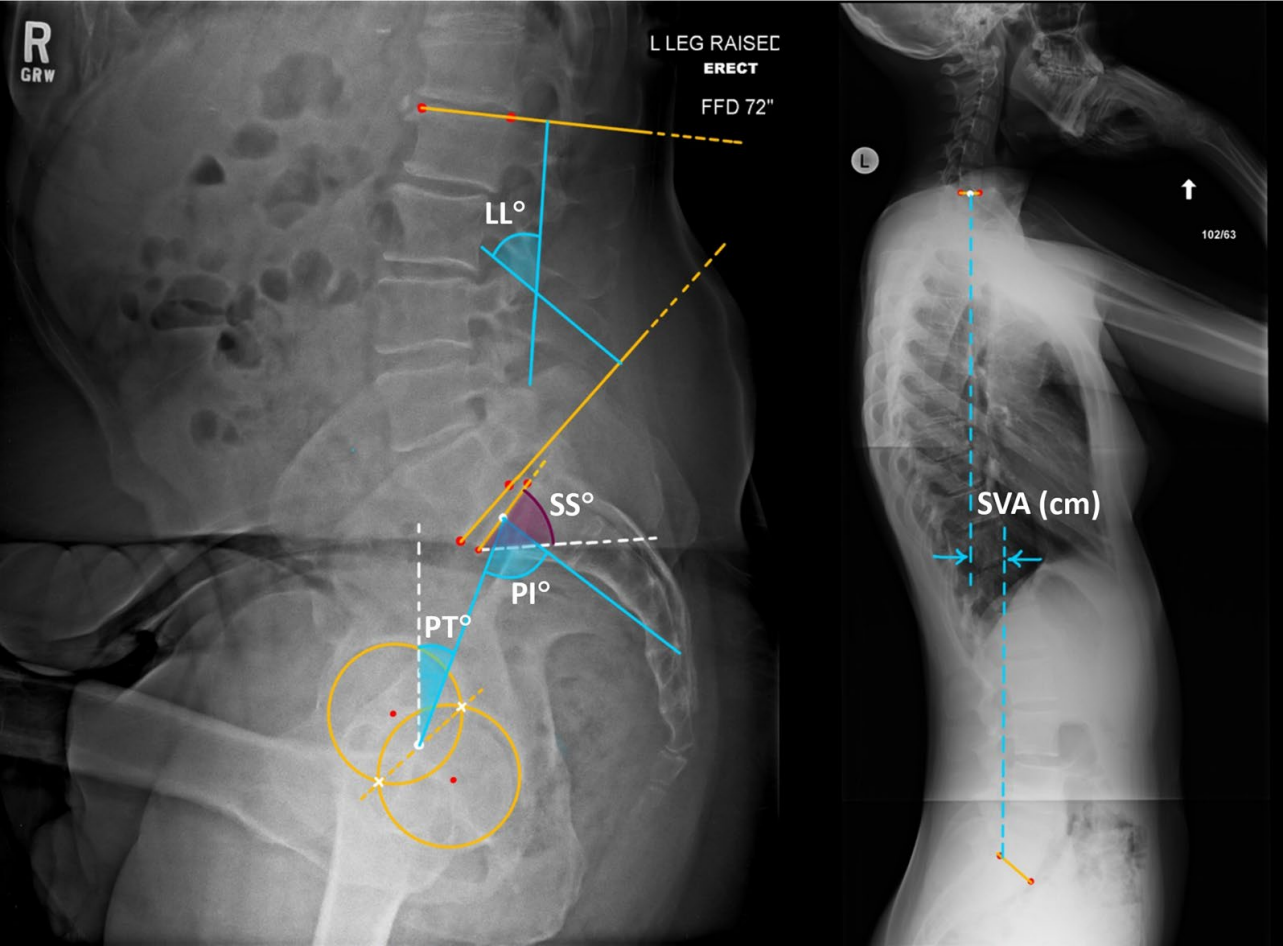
Main spinopelvic measures in lateral X-ray images, considering both femoral heads to calculate the center of rotation for the pelvis
